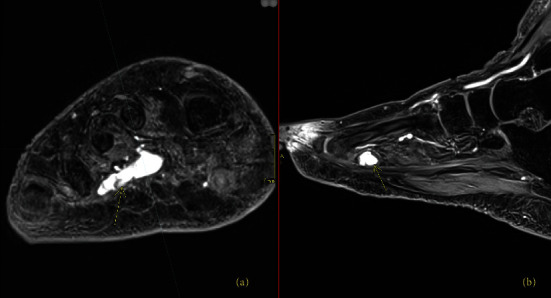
Right foot MRI with contrast for Case 2. Contrast-enhanced axial (a) and sagittal (b) images from an MRI of the foot from patient 2, showing an avidly enhancing soft tissue mass in the plantar aspect of the right midfoot, corresponding to the findings from the DOTATATE-PET/CT in Figure 4.
